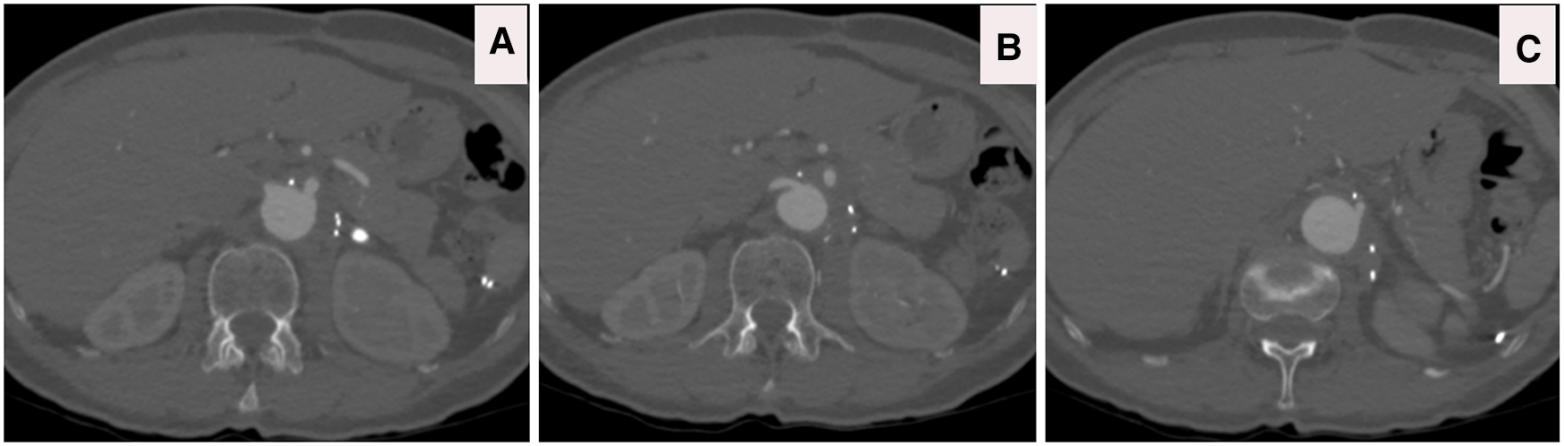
(A–C) CTA axial views four years post sTaged hybrId sinGle lumEn reconstruction (TIGER) without any evidence of aneurysmal diseases or dissection or stenosis; patients are off all of her antihypertensive medications.
